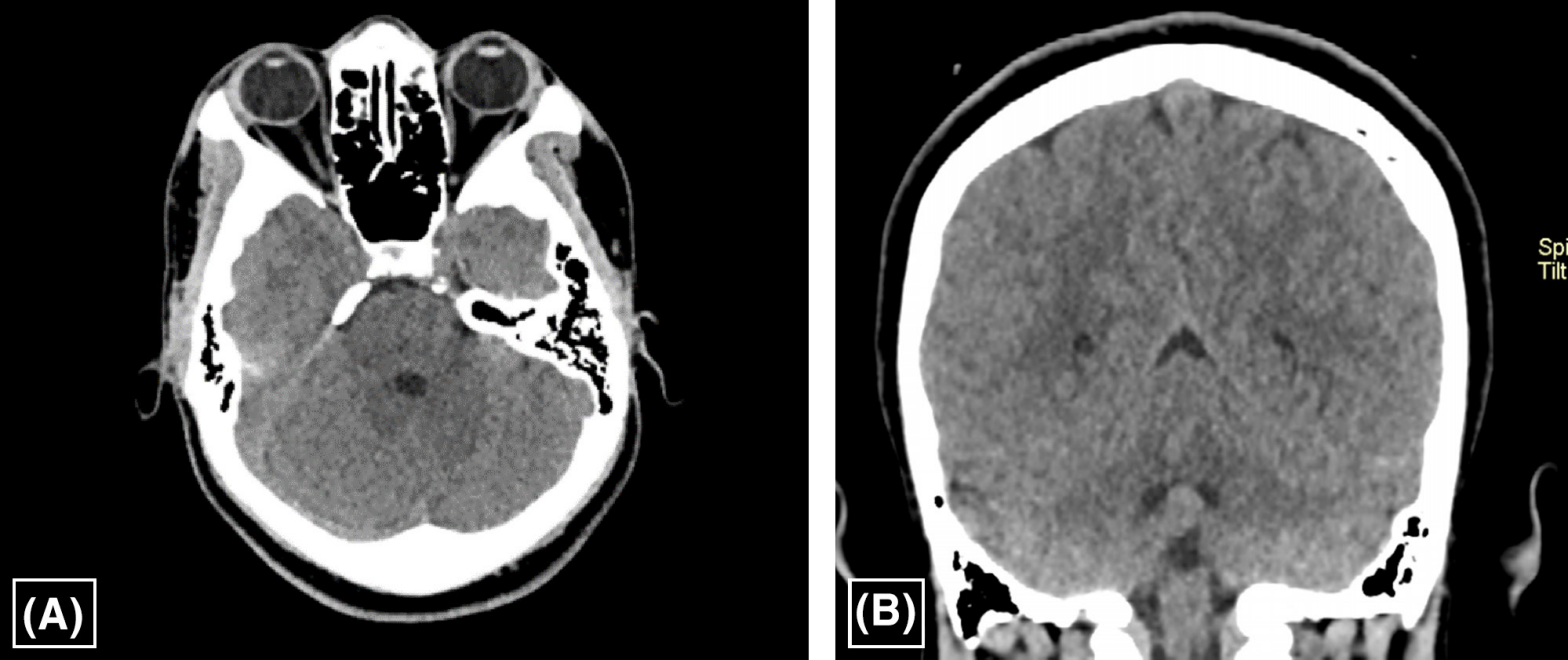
(A) Non‐enhanced axial brain CT at the level of orbits, images show: Completely resolved subperiosteal mass‐like lesion in the left retrobulbar‐extraconal region which is compatible with resolved intraorbital hematoma. (B) Non‐enhanced coronal brain CT at the level of the cerebellum, images show: Completely resolved right extra‐calvarial temporo‐parietal cephalohematoma, with no underlying skull fractures.
